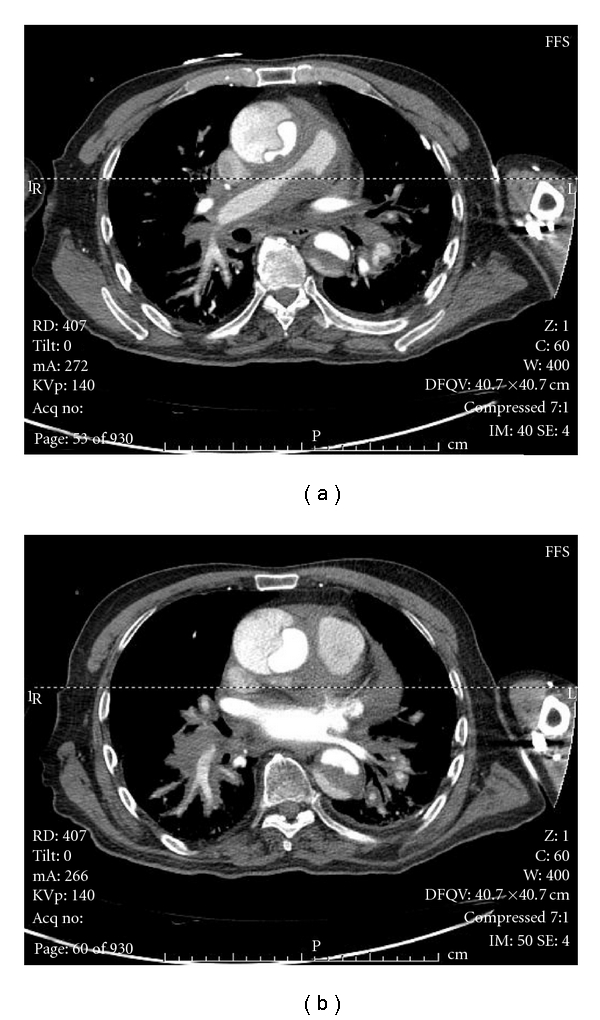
CT scans demonstrate an aortic aneurysm, showing contrast within the true lumen, a pericardial effusion, and haemorrhage extending down the pulmonary arteries and veins to subsegmental branches.
